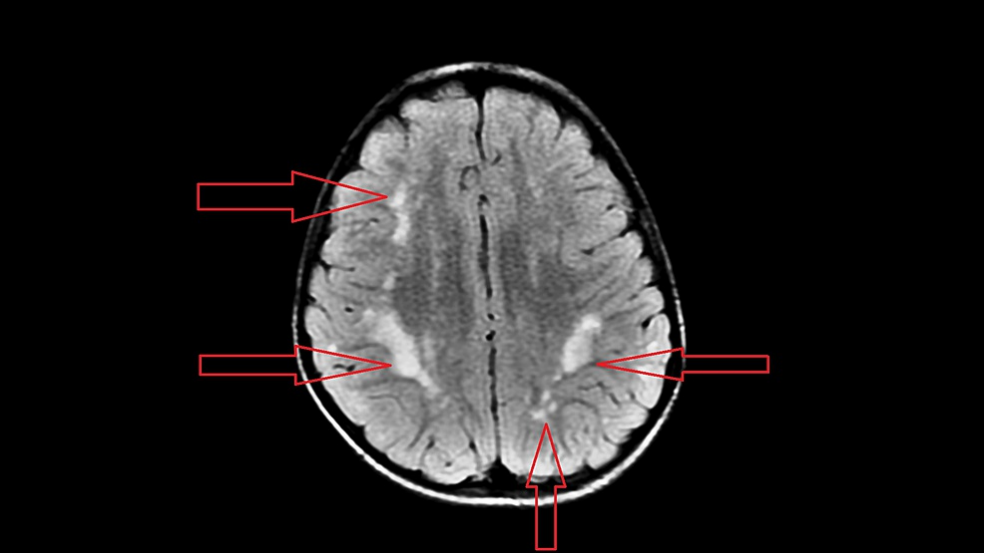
Axial T2 FLAIR image corresponding to T2W image in Figure 1. FLAIR: fluid-attenuated inversion recovery.
